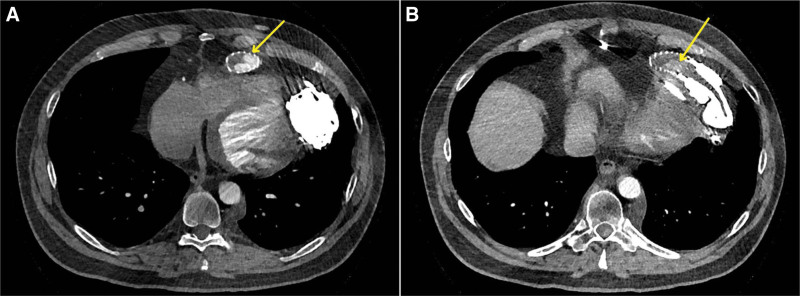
A: A CT angiography image showing a horizontal section of the outflow graft with stenosis due to biological material accumulation between the graft and the bend relief. B: Sagittal section of the outflow graft showing partial luminal stenosis. CT, Computed tomography.
